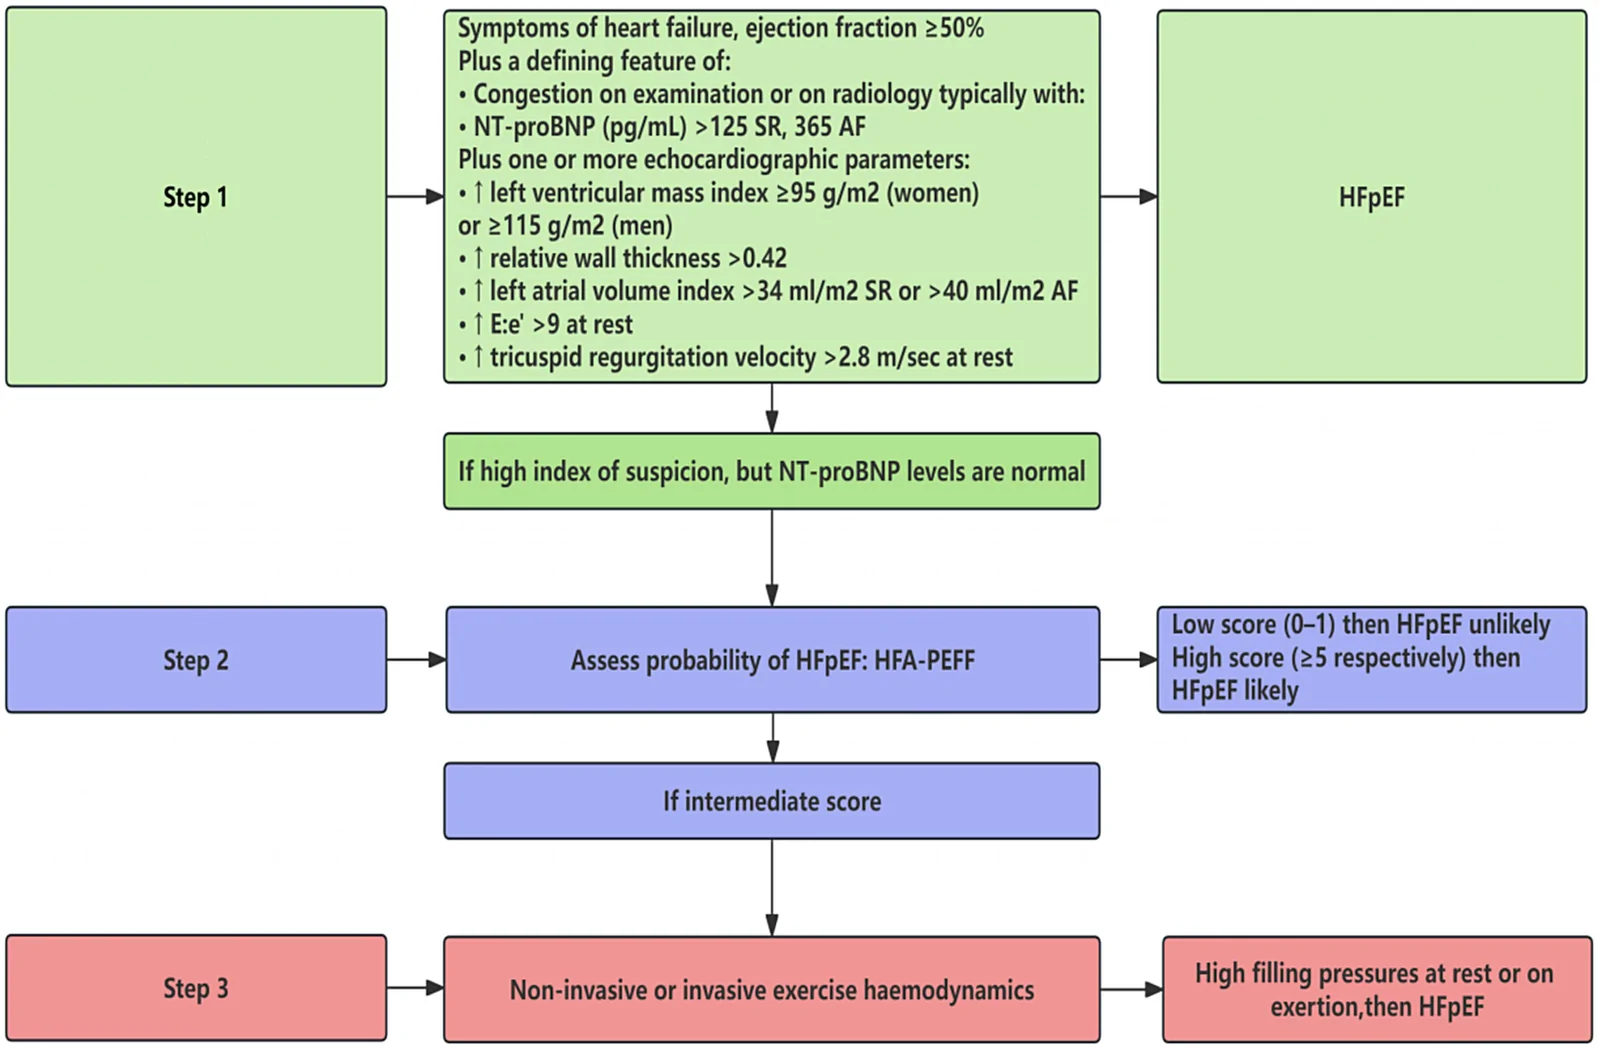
HFpEF diagnostic algorithm. AF, atrial fibrillation; E:e', early diastolic mitral inflow velocity to early diastolic mitral annulus velocity; HFA-PEFF, Heart Failure Association Pre-test assessment, Echocardiography and natriuretic peptide, Functional testing, Final aetiology; HFpEF, heart failure with preserved ejection fraction; NT-proBNP, N-terminal pro-B type natriuretic peptide; SR, sinus rhythm. Reproduced with permission from “HFpEF diagnostic algorithm” by Patricia Campbell, Frans H Rutten, Matthew MY Lee, Nathaniel M Hawkins and Mark C Petrie.
